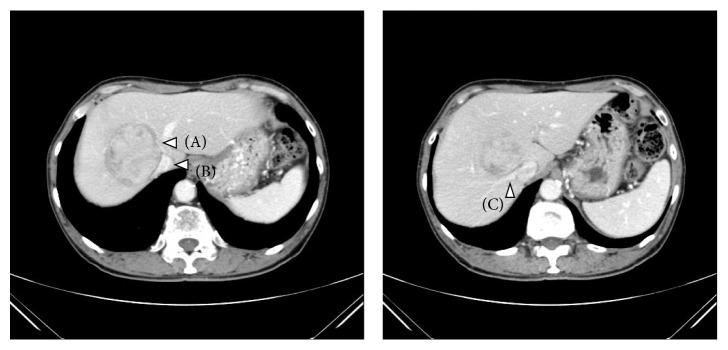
Preoperative CT findings of case number 3 (right anterior sectionectomies combined with middle hepatic vein resection). 58-year-old man with type-C chronic hepatitis developed 7 cm HCC in segment 8–1. The tumor compressed the inferior vena cava (B) and the right hepatic vein (C) widely and also there was an involvement of the middle hepatic vein (A). This patient underwent extended right anterior sectionectomy combined with the middle hepatic vein resection at its root. This case is one of the most complicated cases for pure laparoscopic liver resection due to the long dissection of the inferior vena cava to the right hepatic vein and the resection of the middle hepatic vein at its root.
